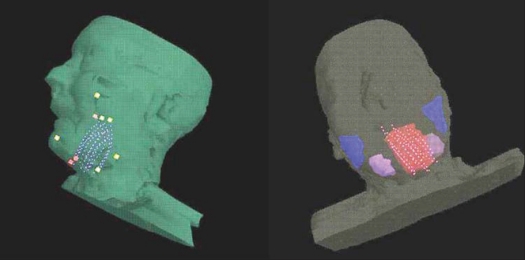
The 3D distribution of reconstructed implant geometry transformed on to the CT dataset
